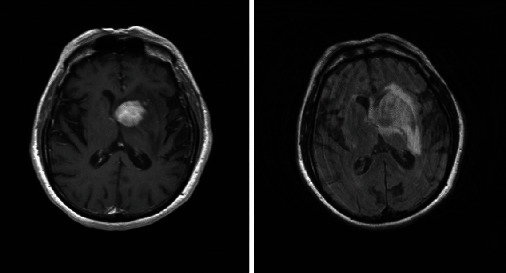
Left medial basal ganglia involving the ependyma of the left frontal horn and right temporal lobe along the course of the right temporal horn with surrounding edema. Radiographic differential diagnosis: PCNSL.
